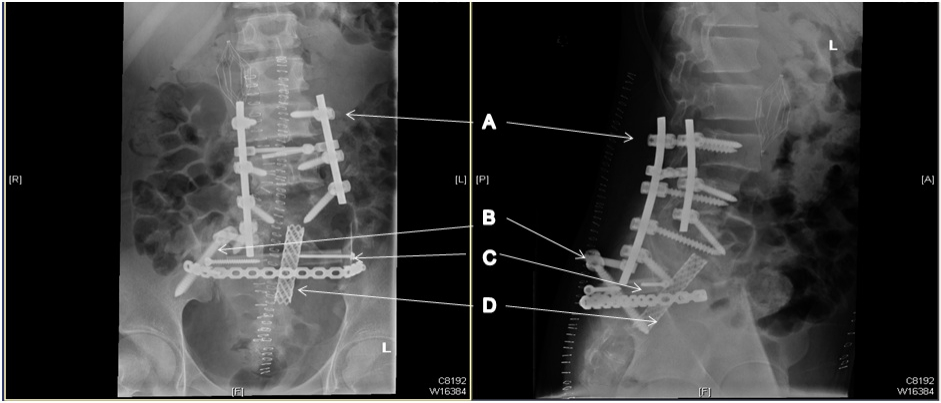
Postoperative MRI showing the post-resection and fusion spinal column. (A) Pedicle screw; (B) Iliac screw; (C) Fibular graft; (D) Strut.
